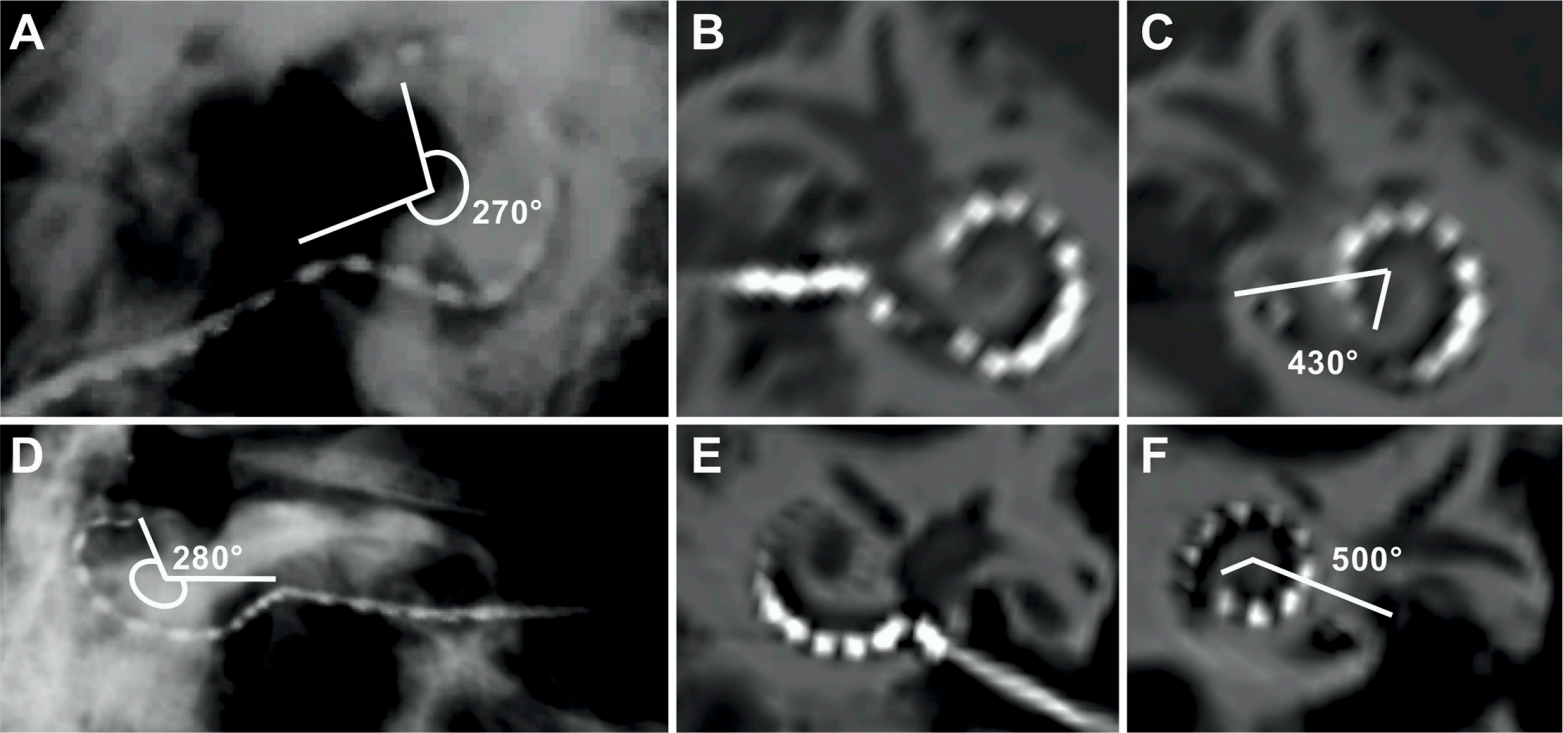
Insertion depth and angle of the first insertion and later insertion with both electrodes: A: X-Ray in Stenver’s view after partial insertion of Electrode-1; B: CT-scan after full insertion, showing an insertion angle of 430° for Electrode-1 (C); D: X-Ray in Stenver’s view after partial insertion of Electrode-2; E: CT-scan after full insertion, showing an insertion angle of 500° for Electrode-2 (F).
